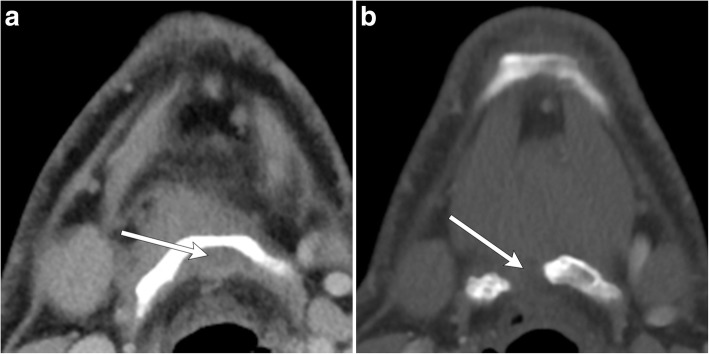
Sistrunk procedure. a Axial contrast-enhanced CT image demonstrates a thyroglossal duct cyst wrapping around the hyoid bone (arrow). b Post-operative axial contrast-enhanced CT image demonstrates removal of the thyroglossal duct cyst and a partial hyoidectomy (arrow)
